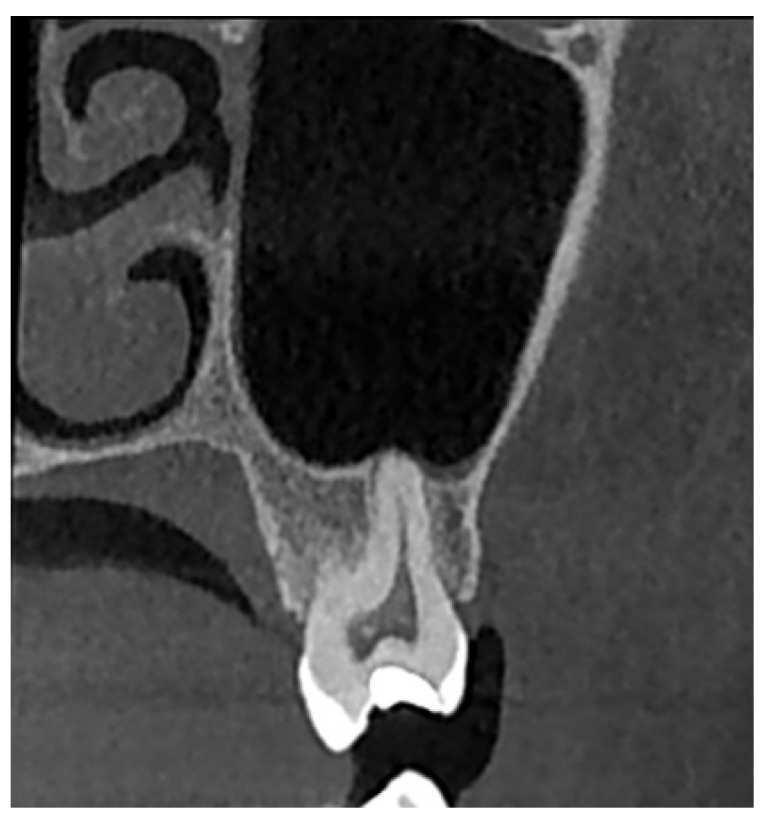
Cross-sectional CBCT reformat along the long axis of tooth 27. Penetrating-type relationship between tooth 27 and the maxillary sinus.
